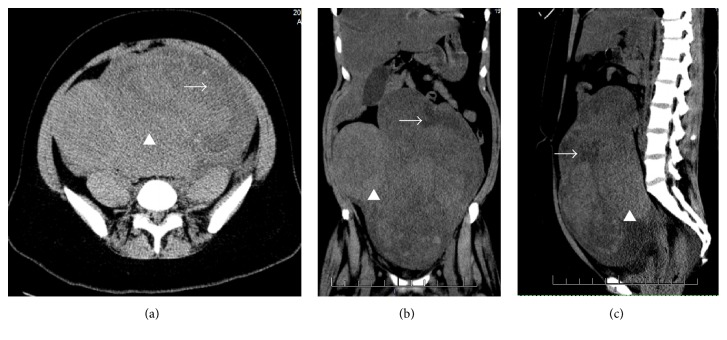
Computed tomography scan of the abdomen and pelvis without contrast showing the pelvic mass with corresponding solid (arrowhead) and cystic components (arrow). Axial (a), coronal (b), and sagittal (c) views.
